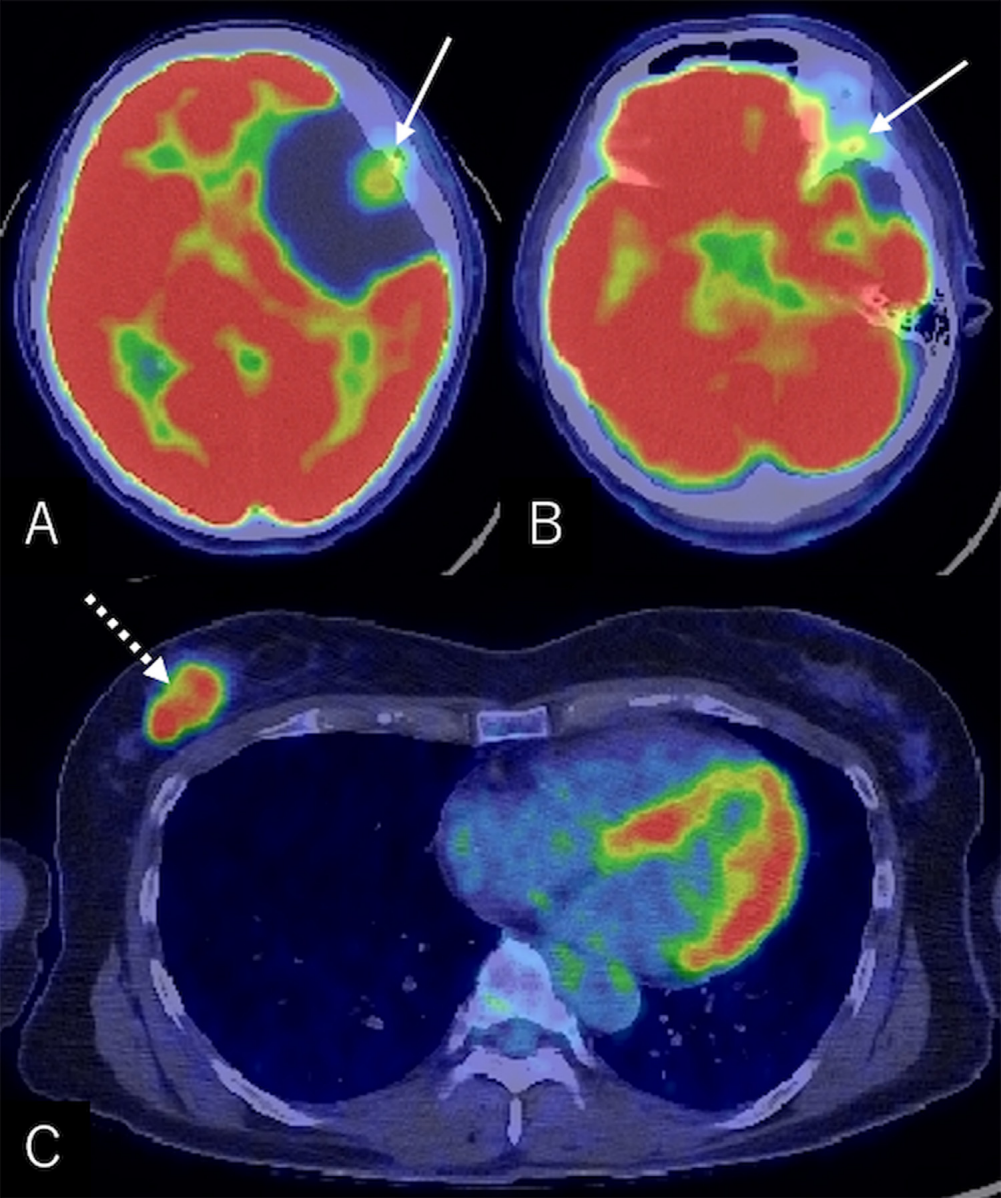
(A–C) Axial F-18 fluorodeoxyglucose positron emission tomography/computed tomography scans showing abnormal, but less considerable accumulations in the intracranial tumor and adjacent frontal and sphenoid bones (A and B*,* arrow) compared to the right breast cancer (C*,* dashed arrow).
